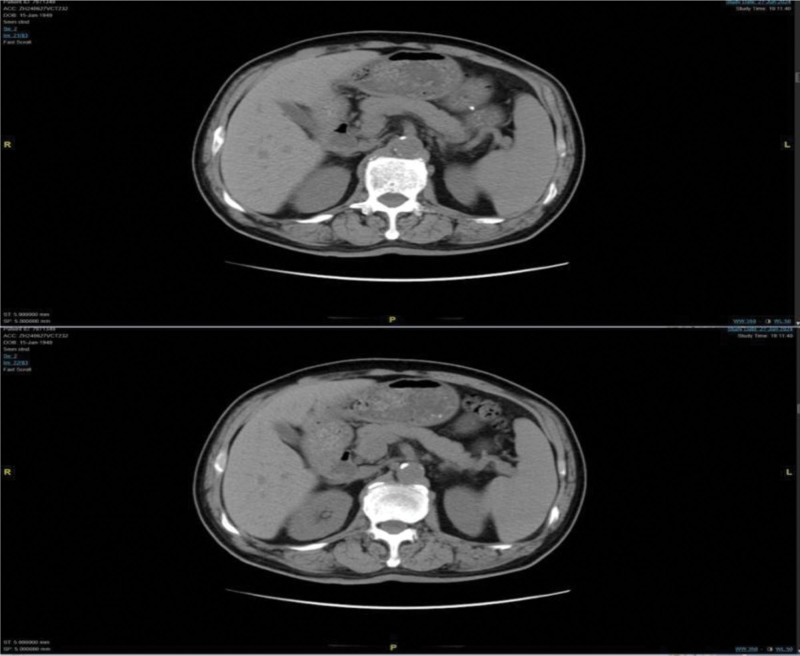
Abdominal CT showing no inflammatory or pathological changes in the pancreas. CT = computed tomography.
